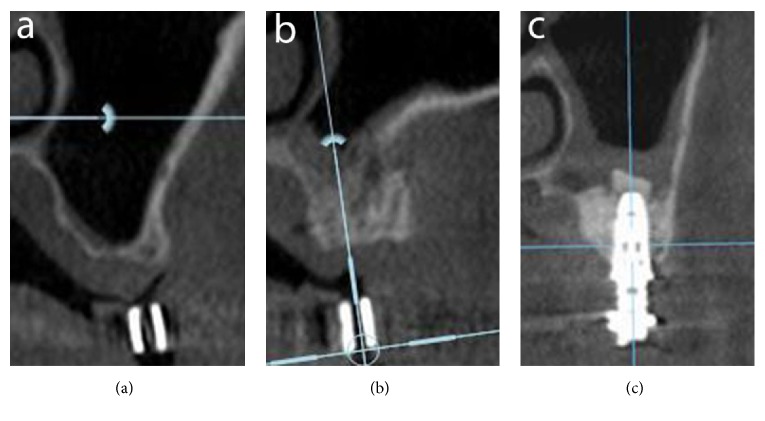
Radiographic evaluation: (a) CT scan before surgery; (b) CT scan after 6 months of graft healing; (c) CT scan after implant placement.
